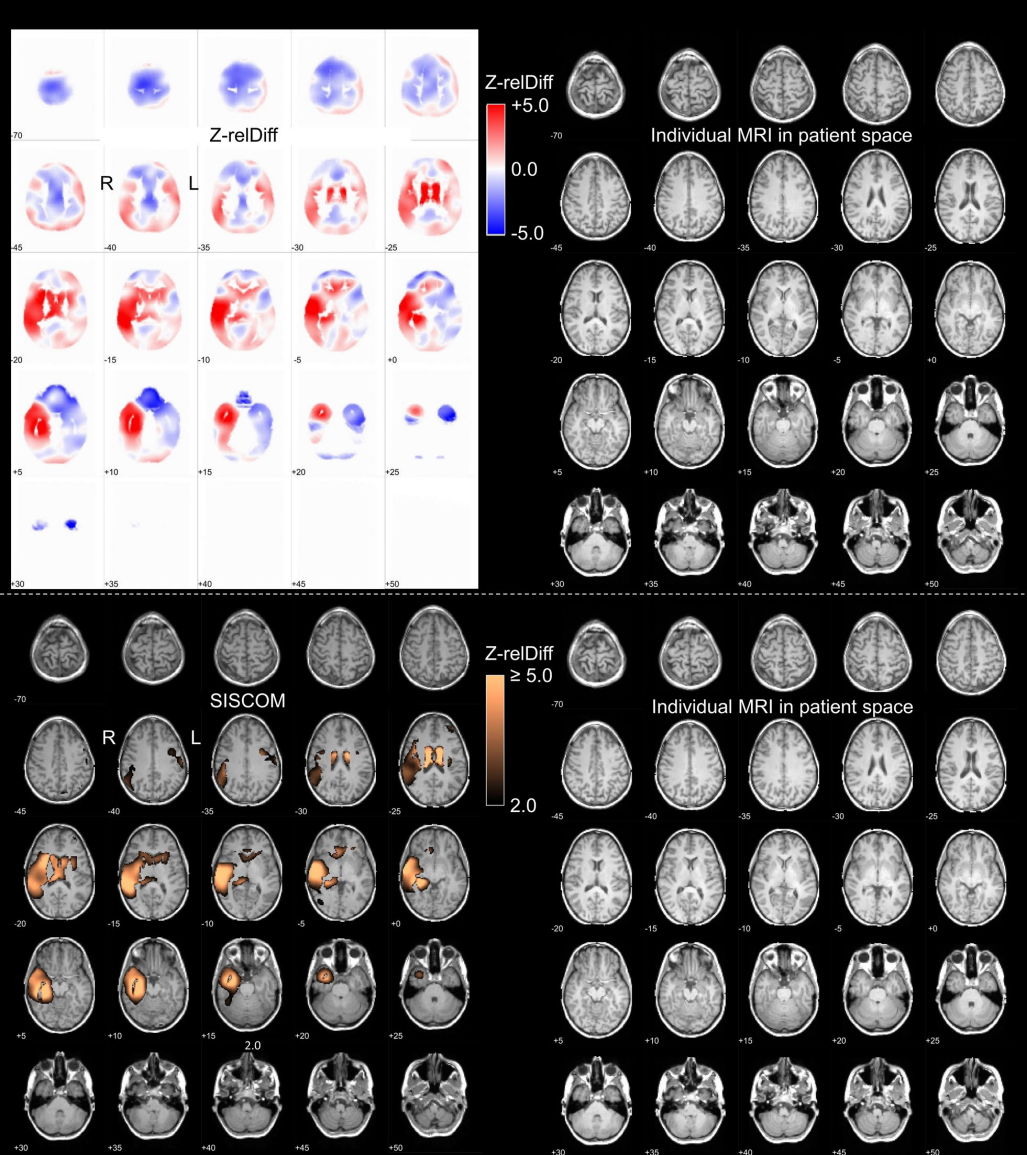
Standardized display for visual reading of SISCOM results in the “full” setting. The upper and lower parts were provided as page 7 and page 8 of an 8-page pdf-document. On page 7, the left side shows the full (non-thresholded) z-relDiff map coregistered to the individual T1-weighted MRI shown on the right. The z-relDiff map is displayed with a split color table to support the discrimination between positive (ictal > interictal) and negative (interictal > ictal) z-scores. On page 8, the left side shows the SISCOM map thresholded at z ≥ 2.0, the right side again shows the individual T1-weighted MRI. The example images are from the same patient as in Figs. 1, 2
